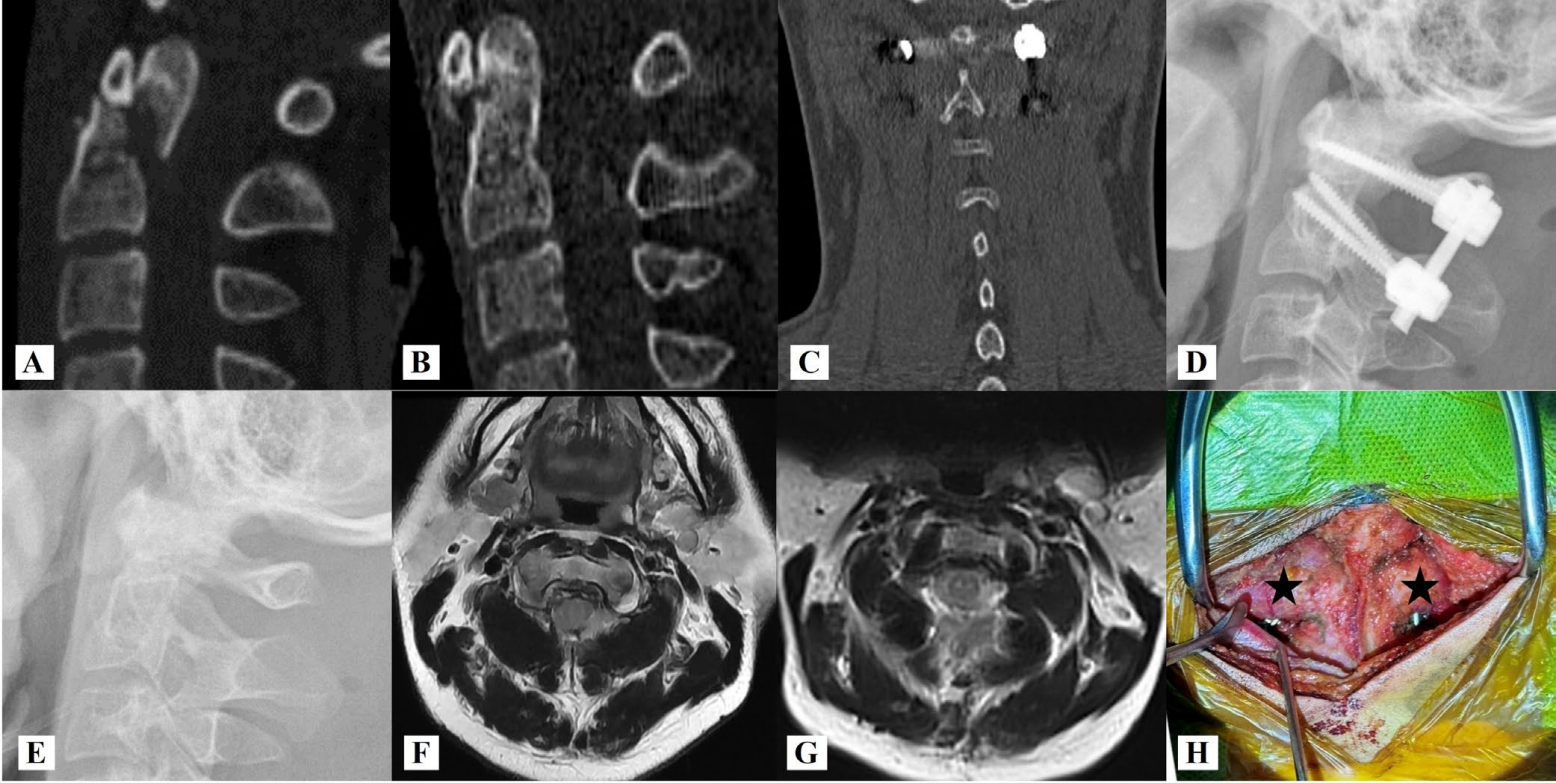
Male, 25 years old, injured in car accident, Grauer type II_B_ fracture treated by the MNLA. (A) Preoperative sagittal view of CT scan shows odontoid fracture combined with dislocation; (B) postoperative CT scan shows fracture was reset; (C) postoperative coronal view of CT scan shows the preserved signal of the muscles connected to the spinous process of C2; (D) postoperative lateral X‐ray image indicates that the fracture has been successfully realigned; (E) lateral X‐ray imaging of the upper cervical spine following the removal of the internal fixator revealed signs of odontoid healing; (F, G) axial views of MRI before surgery and 3rd days post‐operation; (H) posterior view of the surgical site after locking the screw‐rod system, the black star indicates the OCI.
